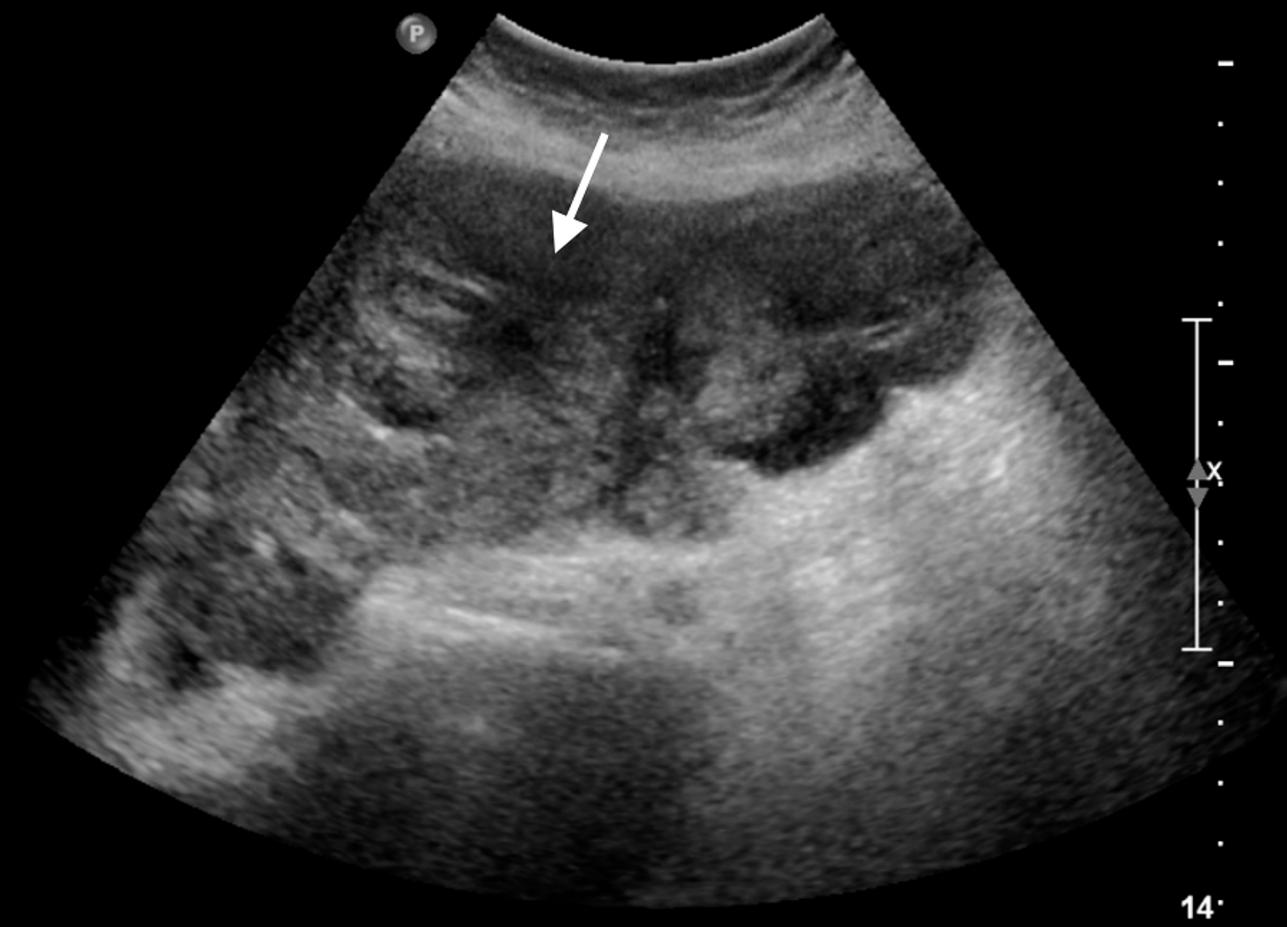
Liver ultrasound showing innumerable hepatic lesions concerning for malignancy
